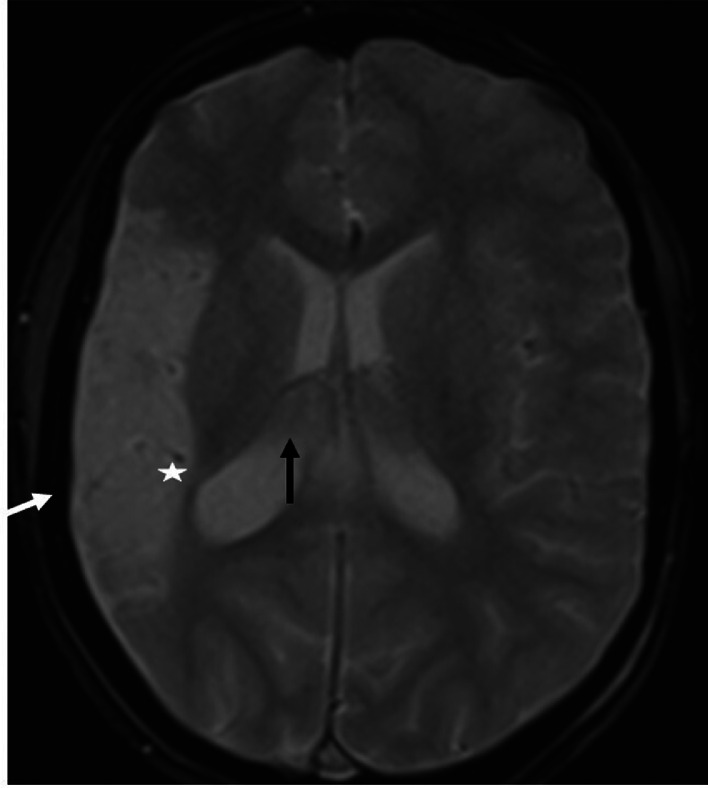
Axial T2* MRI in Sturge-Weber syndrome type III The sequence reveals dilatation of transparenchymal veins (*) with enlarged ipsilateral choroid plexus (black arrow) and calvarial thickening (white arrow). These features represent the characteristic parenchymal pattern of SWS type III.
